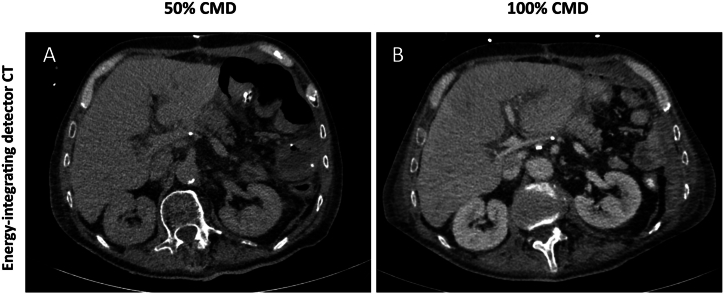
Representative axial images (window width/window level: 350/60 HU) of the same patient with 50% contrast media dose (CMD) (A) and 100% CMD (B) acquired with an energy-integrating detector CT (EID-CT). The images with 50% CMD were considered non-diagnostic.
